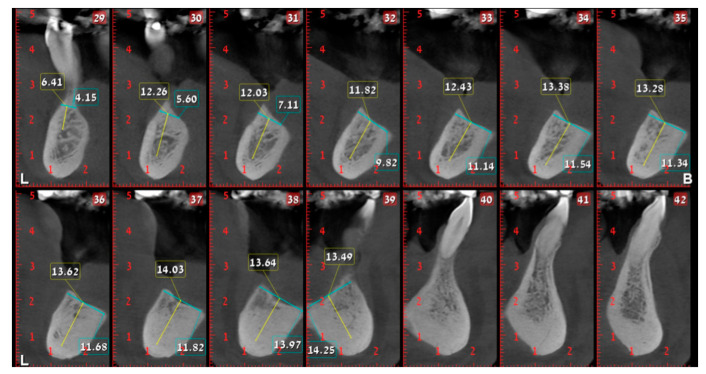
Two cm vertical defect in anterior mandibular alveolar ridge in CBCT imaging; cross-sections of reconstruction.
